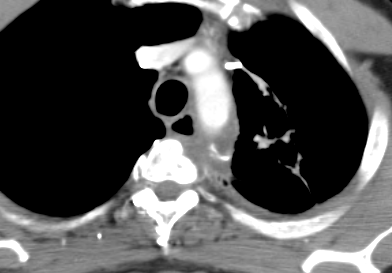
Computed tomographic scan obtained 10 months after treatment.
